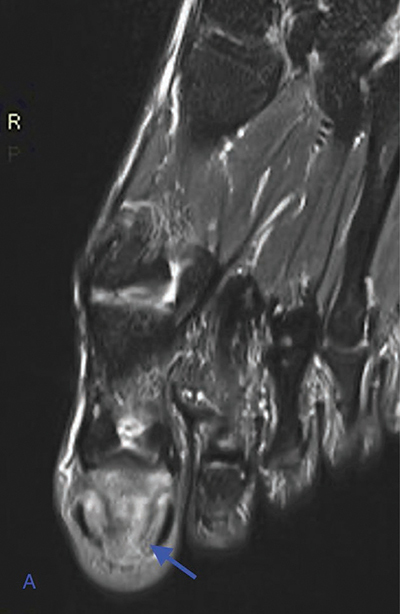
Axial FS T2-WI MRI shows bone marrow edema at the distal phalanx (arrow).
